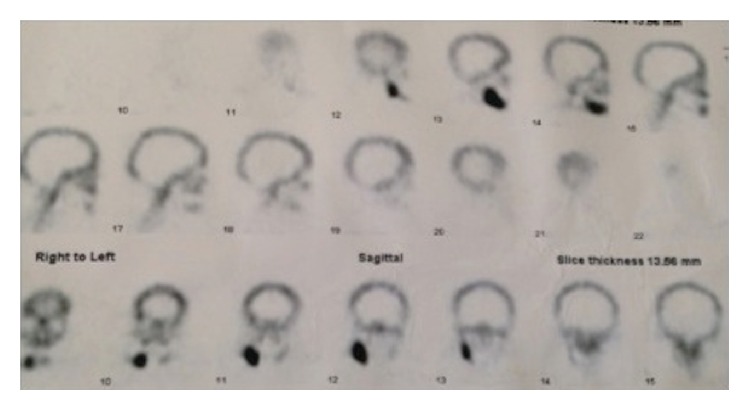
Static whole body bone scintigraphy performed on October, 2013, revealed an intensive trace uptake at the right mandible.
